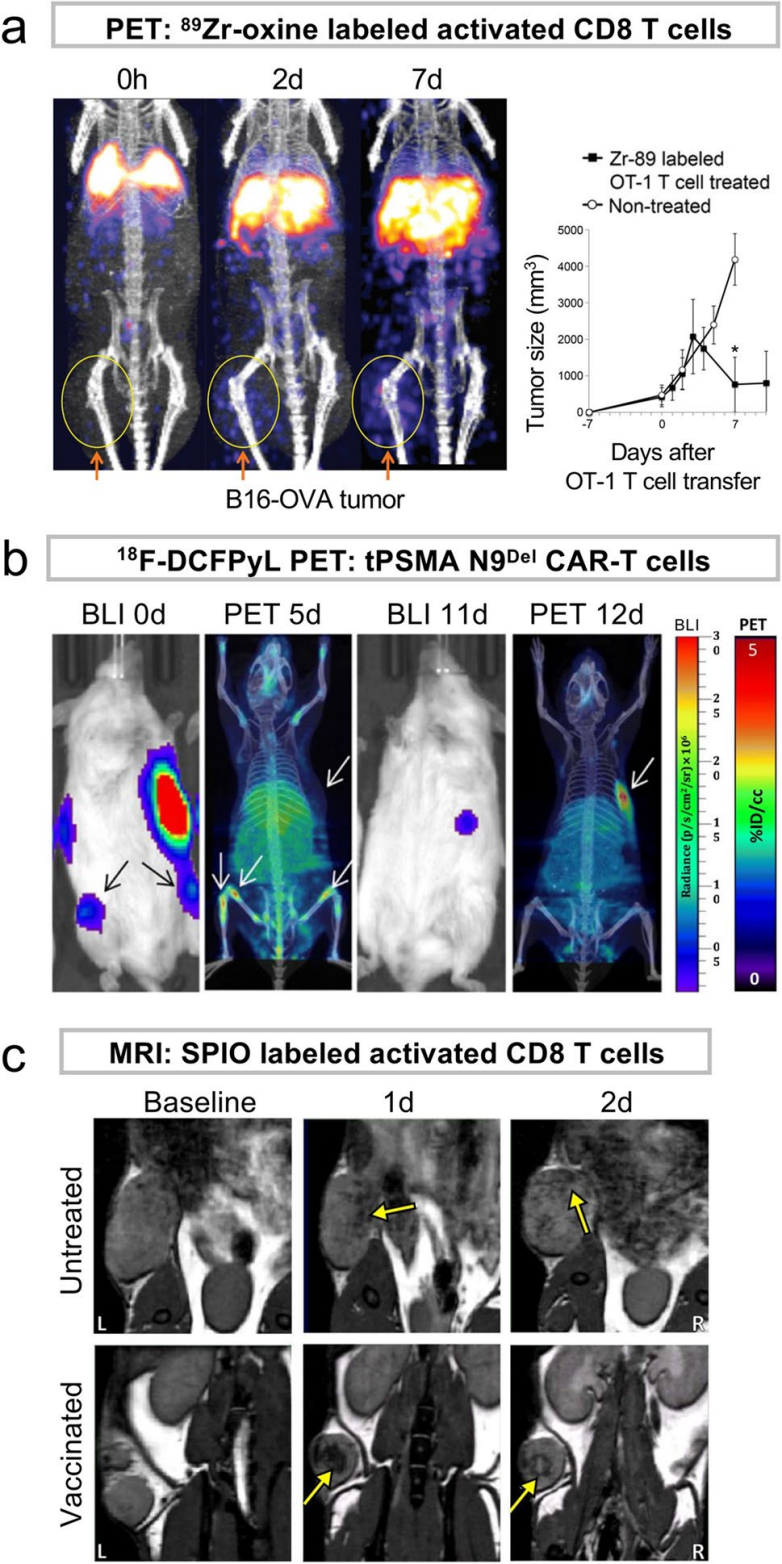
PET and MRI detection of ex vivo labeled and indirectly labeled adoptively transferred T cells targeting cancers. a PET/CT images of ^89^Zr-oxine-labeled OT-1 CD8 T cells accumulating in the B16-OVA melanoma tumor, which induced tumor regression. Adapted from [17]. b
